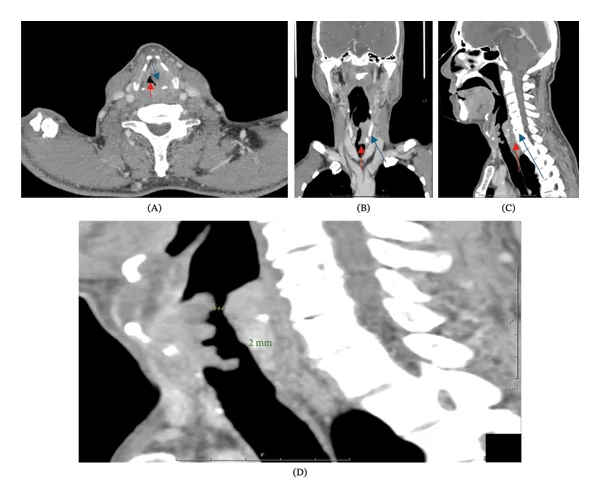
Preoperative contrast‐enhanced CT images of the neck. (A) Axial, (B) coronal, and (C) sagittal views demonstrating a transglottic laryngeal tumor. Red arrows indicate the markedly narrowed residual airway lumen, whereas blue arrows indicate the transglottic tumor. (D) Magnified sagittal CT image demonstrating the narrowest residual airway lumen, measuring approximately 2 mm.
